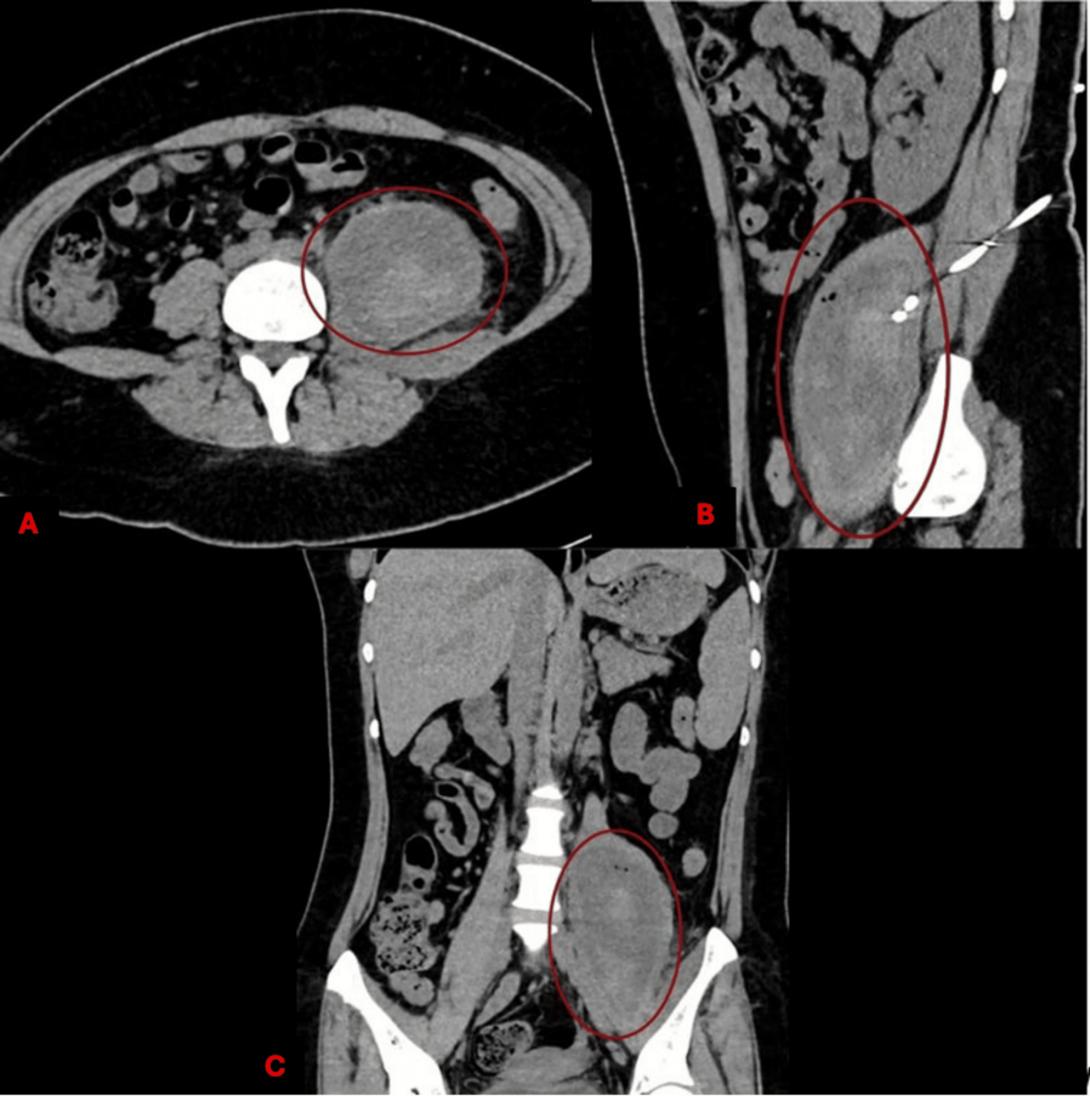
Hematoma in the iliopsoas noticed via contrast-enhanced computed tomography (CT) in a bone window during the three-month follow up. The CT scan shows a decrease in the initial volume to 257 cc. Cross-section (A) and sagittal section (B) show the correct placement of drainage in the hematoma. Pane C showcases the coronal section. Cc: cubic centimeters
